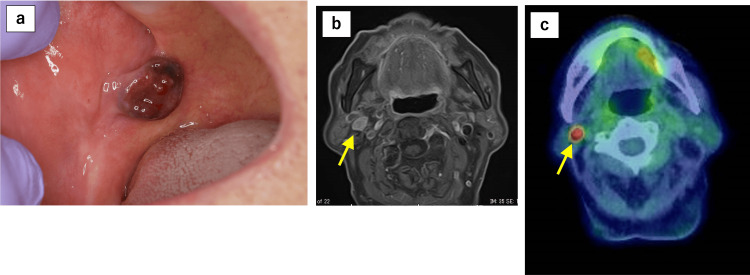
Initial imaging findings of buccal mucosal malignant melanoma and cervical lymph node metastasis (a) A polypoid mass is observed on the right buccal mucosa, and buccal mucosal malignant melanoma is diagnosed by biopsy. Right cervical lymph node metastasis is detected on (b) MRI (gadolinium-enhanced T1-weighted imaging with fat suppression) and (c) PET-CT MRI: magnetic resonance imaging; PET-CT: positron emission tomography-computed tomography
